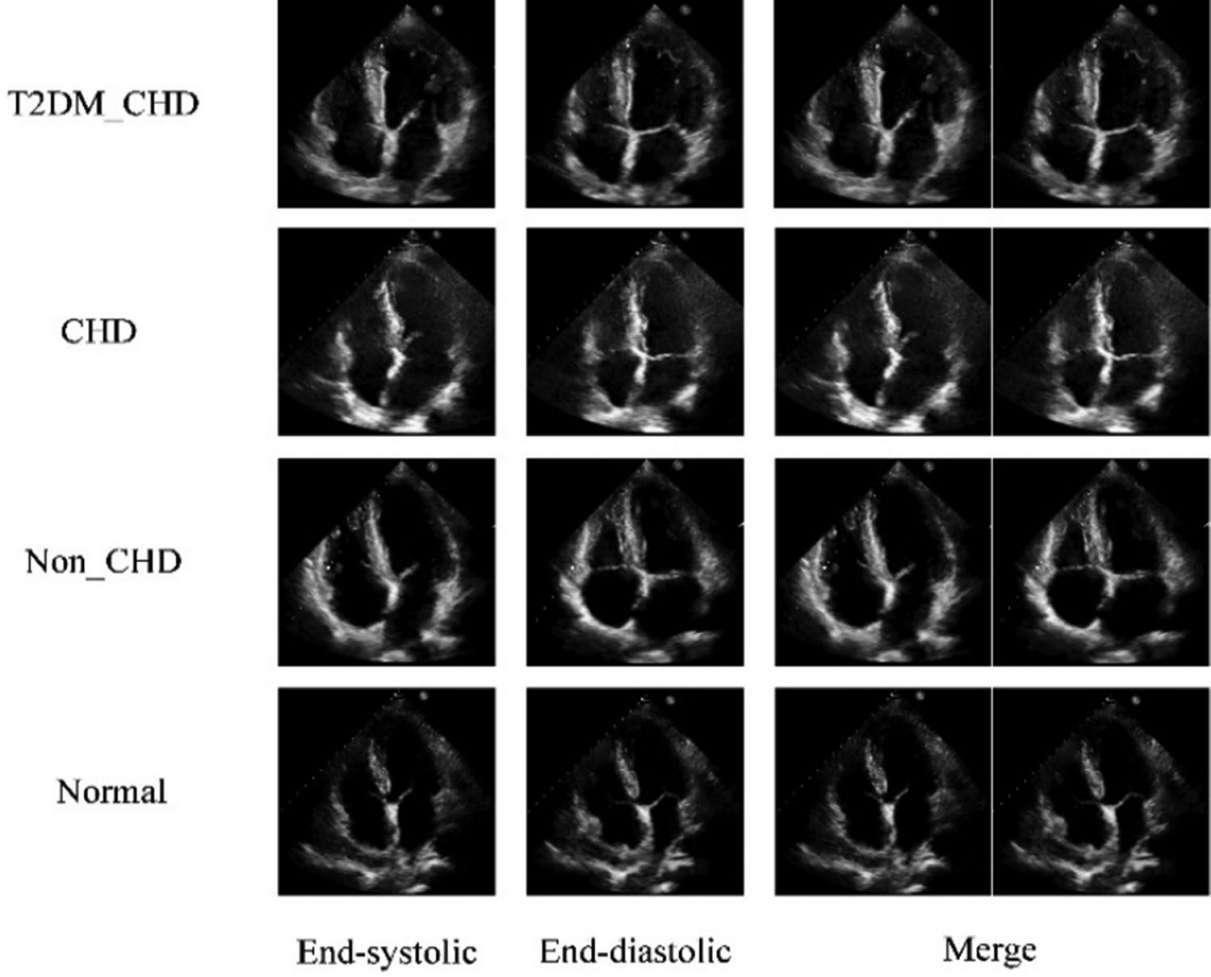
Typical ultrasound images of 4 types of diseases.
